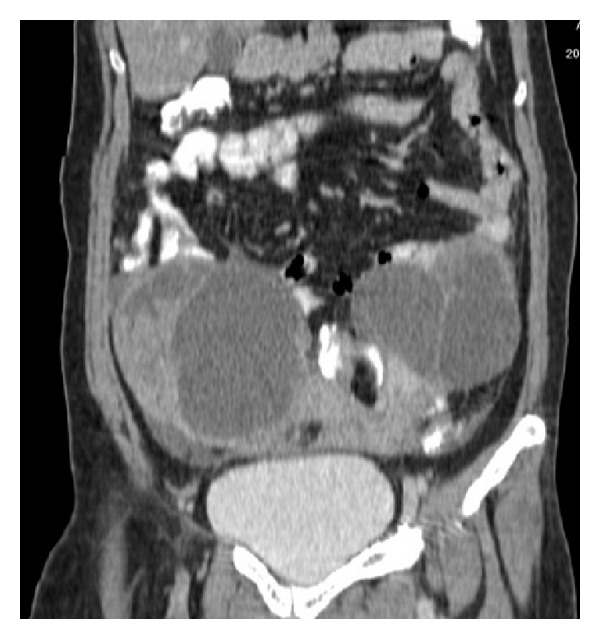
CT scan of the abdomen showing predominantly cystic lesions with internal septations and solid component which appeared after contrast enhancement in bilateral adnexal regions of size 98 × 88 × 107 mm on right side and 110 × 52 × 94 mm on left side.
